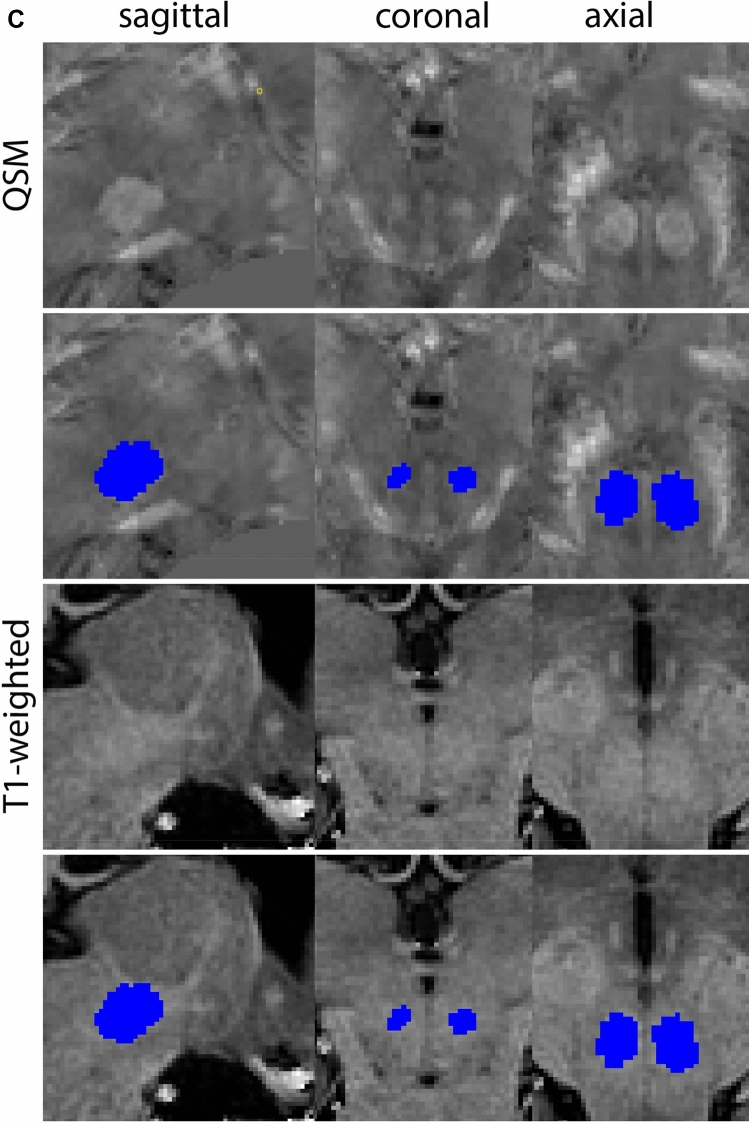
a The red nucleus (RN) at a central level is clearly visible as a rounded structure in all three viewing planes in the QSM contrast. Note the limited visibility of the RN in the T1-weighted contrast. b The red nucleus (RN) at a rostral level can still clearly be identified as is decreased in size. Note the limited visibility of the RN in the T1-weighted contrast. c The red nucleus (RN) at a caudal level can still clearly be identified as is decreased in size. Note the limited visibility of the RN in the T1-weighted contrast
